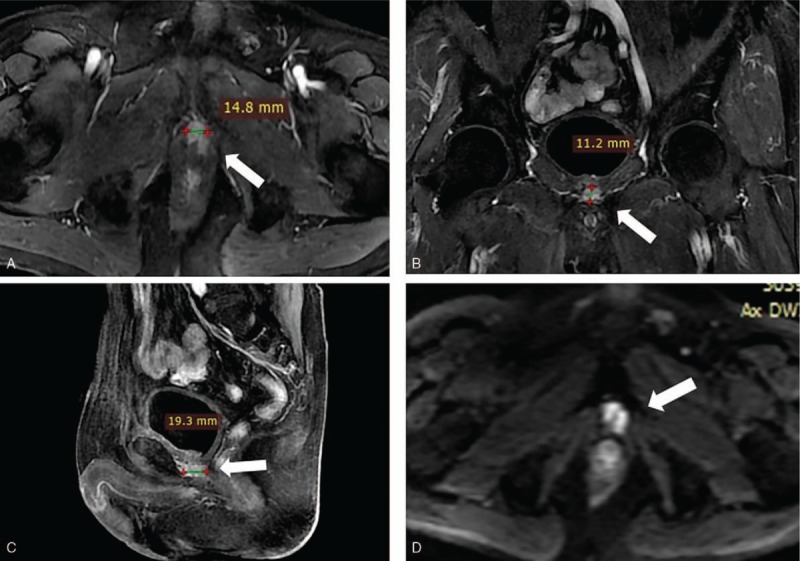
Pelvic MRI (2016-2-22). Follow-up MR images acquired 9 years after initial RP, T2WI MR images (A, B, C) and DW image (D) reveal an irregular nodule (arrow) with high intensity located on resected prostate tumor bed. MRI = magnetic resonance imaging, RP = radical prostatectomy.
